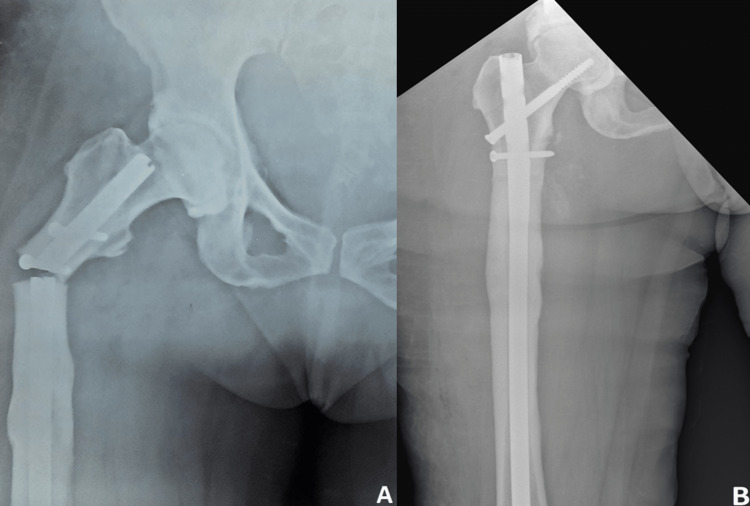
Pre-operative (A) and post-operative (B) one year follow up radiograph of implant failure.
